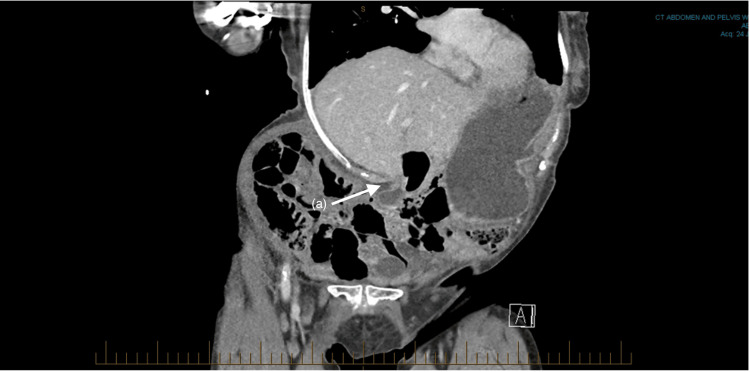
Coronal slice of a CT Abdomen and Pelvis. (a) Gastric outlet obstruction due to impingement by the right chest wall
